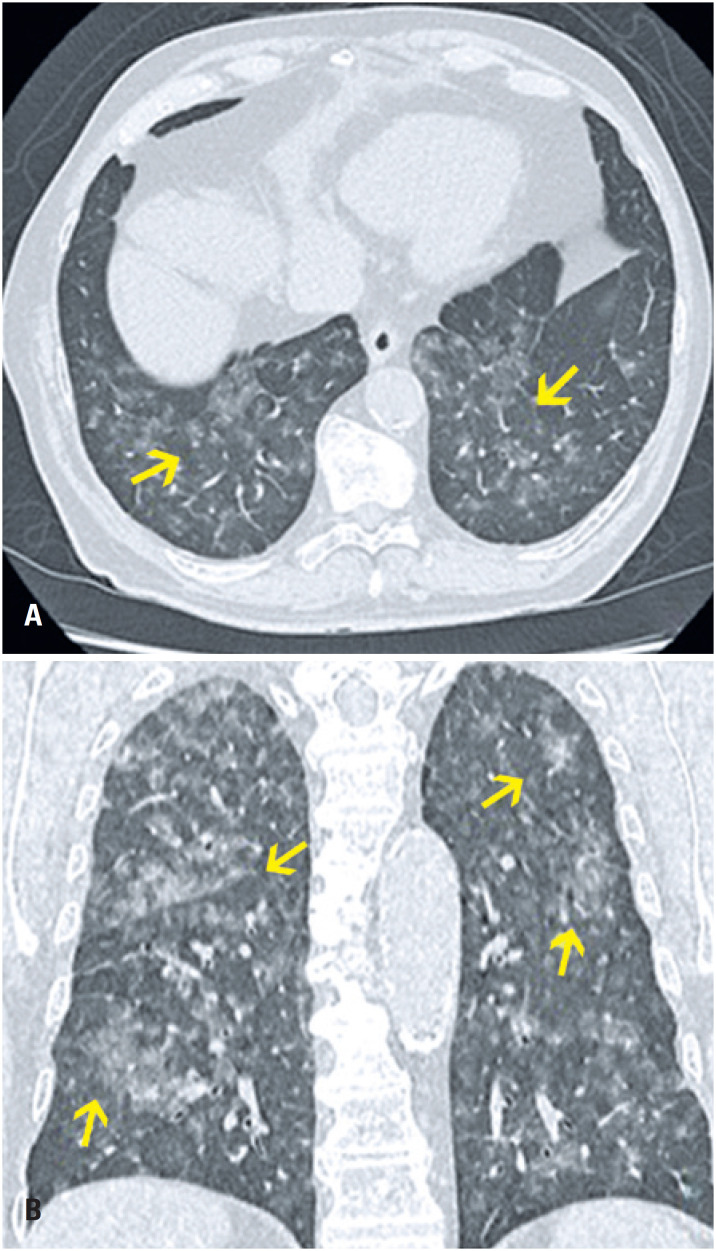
An 85-year-old male patient presented with epistaxis and hematuria for one day. He reported having had recently increased the dose of warfarin. Computed tomography in (A) Axial section and (B) Coronal reformation image: numerous centrilobular ground-glass opacities distributed in all pulmonary lobes
